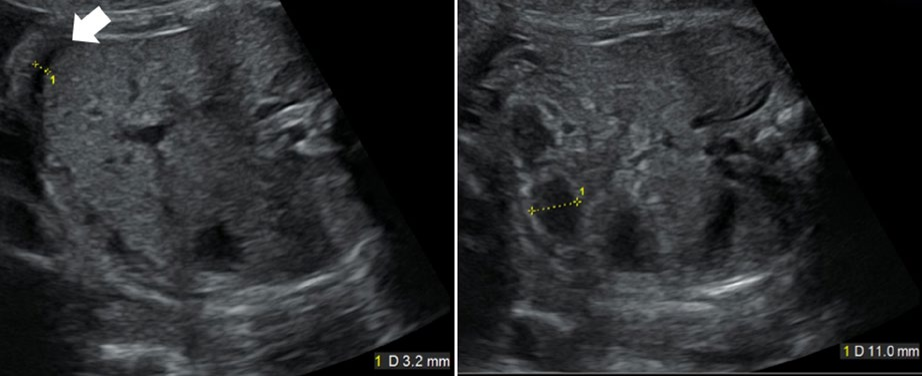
Transverse view of prenatal ultrasound at 31 wk gestation. Right imaging shows mild dilated hyperechogenic transverse colon, and left imaging shows small amount of ascites (arrow)
